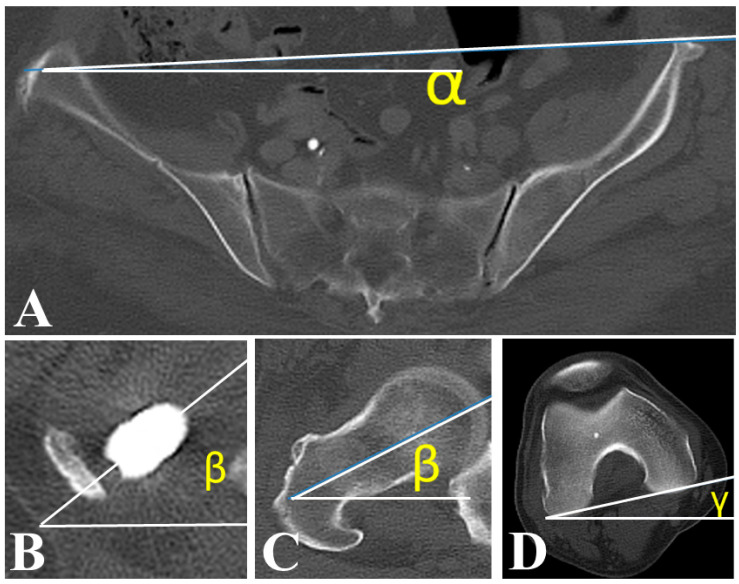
Computed tomography (CT) measurements of femoral rotation angle. (A) Angle α was defined as the angle formed between the CT table line and the line connecting bilateral anterior superior iliac spines. (B,C) Angle β was defined as the angle between the CT table line and the femoral neck axis. (D) Angle γ was defined as the angle between the CT table line and the posterior condylar line. Functional stem anteversion or external rotation angle of the femur was measured by subtracting the value for the rotation angle of the superior anterior iliac spine line from that of the stem angle (β–α) or angle of the posterior femoral condyle axis (γ–α) using a CT image acquired after surgery.
